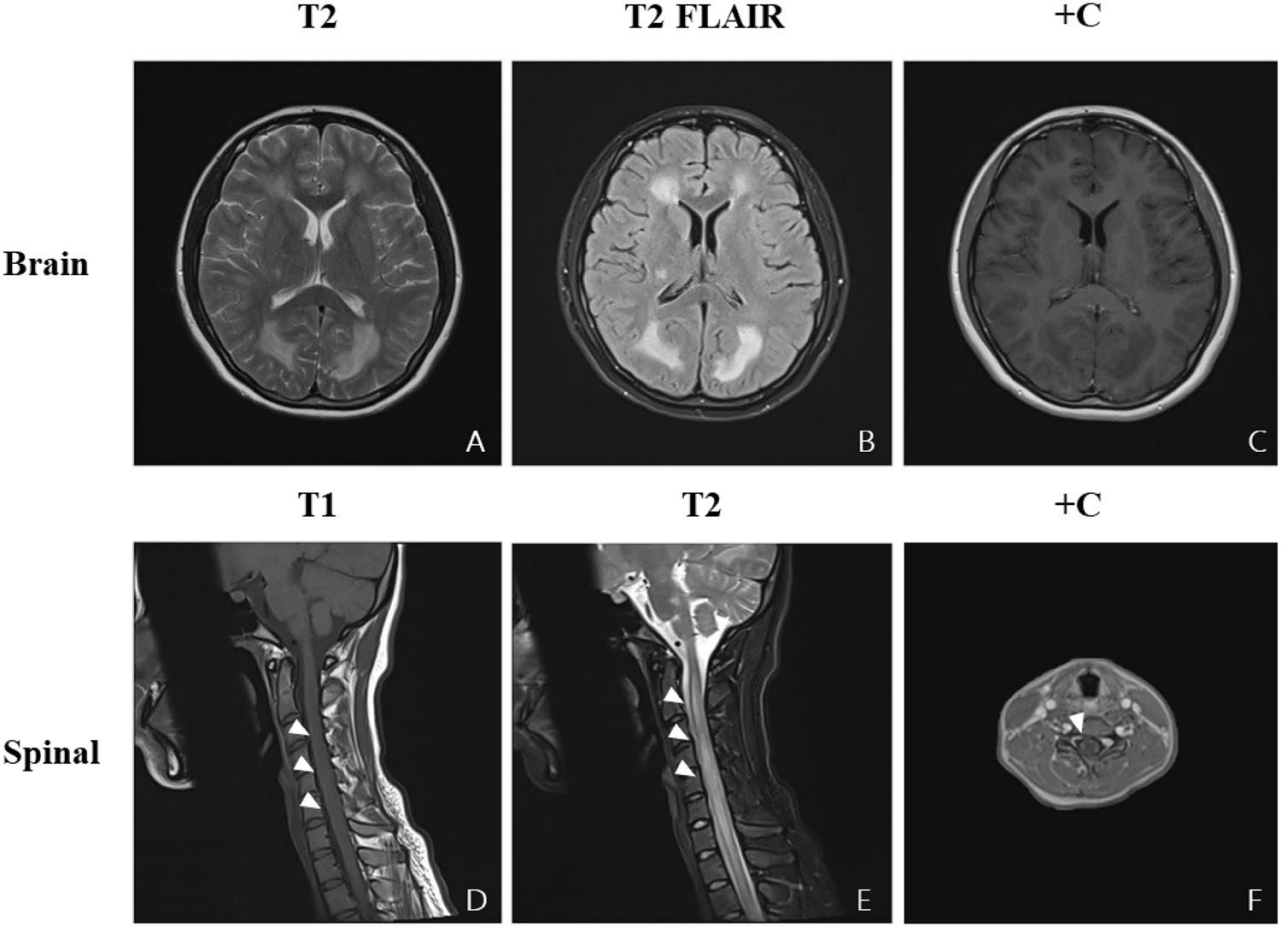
Brain and spinal cord MRI in Patient 5. A–C show the patient’s brain imaging, and D–E show spinal imaging. A, B Hyperintense signal presented in the periventricular white matter on T2 and T2-FLAIR; C no gadolinium enhancement was observed on the brain enhanced scan; D hypointense signal presented along the spinal cord on T1 and hyperintense signal presented in the same lesions on T2 (E); F circular hyperintense signal was found along the edge of spinal cord on enhanced scan
